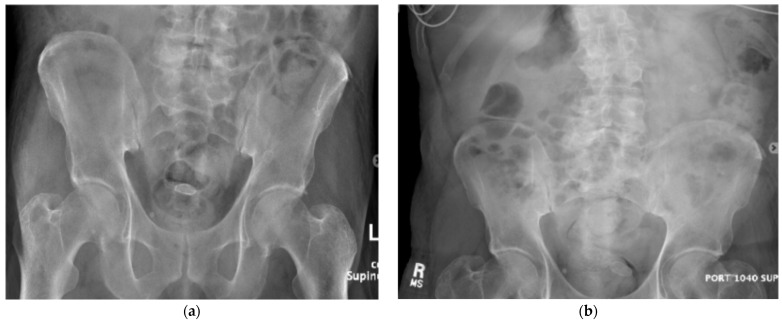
(a,b) Serial abdominal radiographs during hospitalization. (a) Day 8. Supine abdominal radiograph demonstrating moderate fecal retention with gaseous distention of the colon, most prominent in the ascending colon and rectosigmoid region. No radiographic evidence of mechanical bowel obstruction is identified. (b) Day 16. Follow-up abdominal radiograph demonstrating persistent fecal retention and gaseous distention without evidence of mechanical bowel obstruction. An incidental radiopaque density in the left renal region is suggestive of nephrolithiasis. Lumbar levoscoliosis and degenerative changes in the lumbar spine are also noted.
